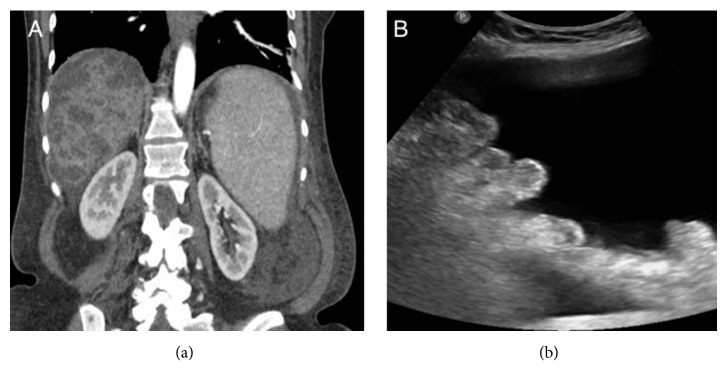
(a) Computed tomography of abdomen and pelvis (coronal view) showing normal architecture and enhancement of bilateral kidneys with no evidence of renal cysts. (b) Abdominal ultrasonographic image showed gross ascites.
